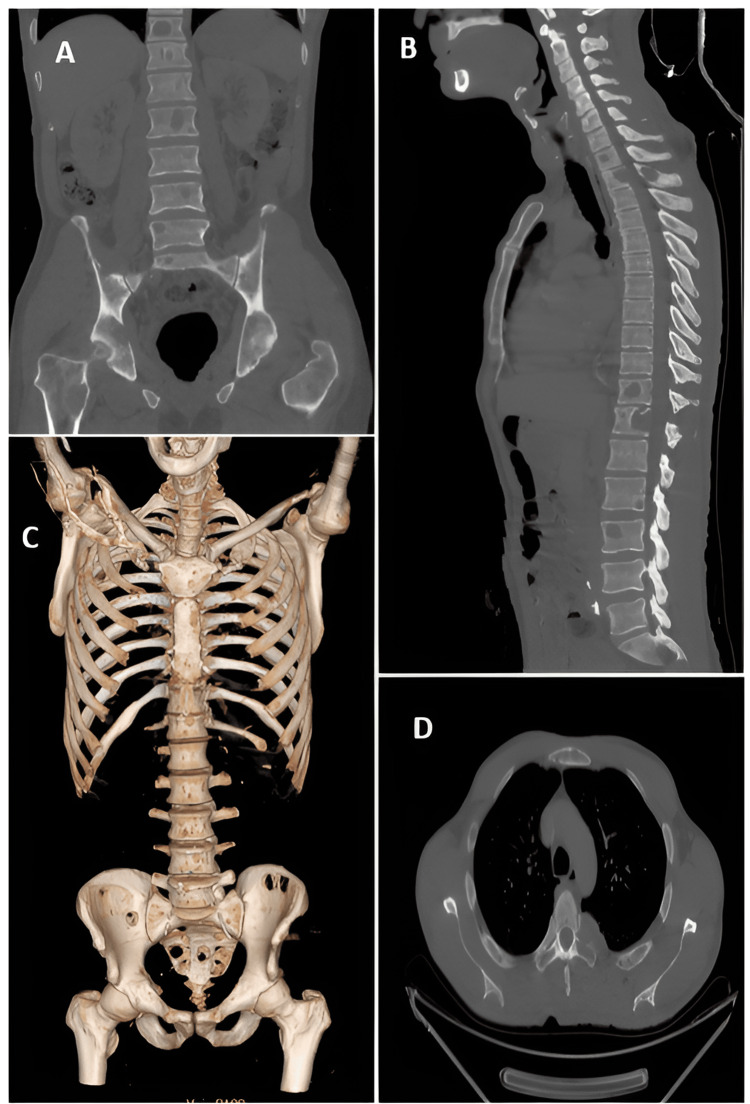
Thoracic-abdominopelvic computed tomography (CT) scan A&B- Sagittal and coronal bone window sections showing multiple lacunar bone lesions in the axial skeleton and pelvis. C- Thoracic-abdominal-pelvic CT scan with bone reconstruction showing the same lesions. D- Axial sections in the bone window: lytic lesions of the costal lacunae and transverse processes.
